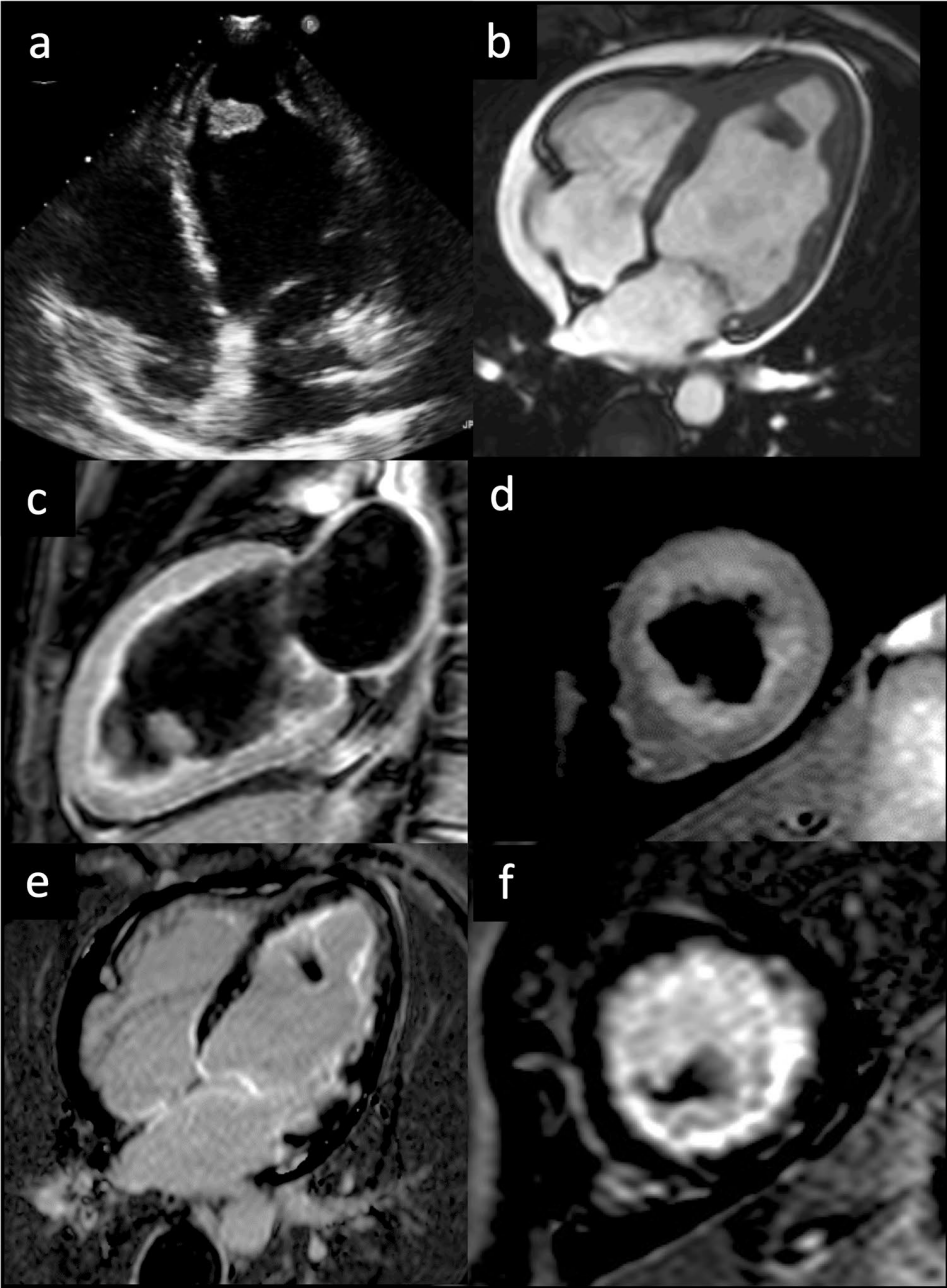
Endomyocardial fibrosis—40-year-old patient with hypereosinophilia. Transthoracic echocardiogram in 4-chamber projection demonstrates the presence of an apical thrombus (a). Cine-SSFP in 4-chamber view (b) confirms echocardiographic findings and evidence a reduction in EF (35%). STIR in 2-chamber plane (c) and short axis (d) shows no signs of edema. LGE images in 4 chambers (e) and short axis (f) demonstrate a diffuse subendocardial hyperintensity and a circumferential pericardial effusion
